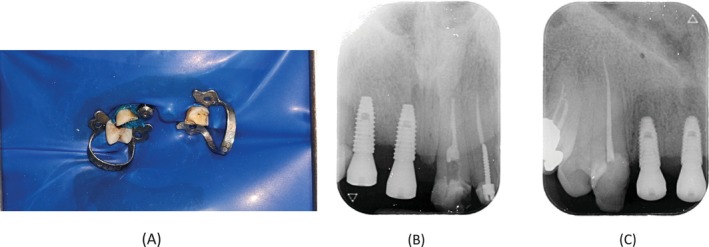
(A) Rubber dam placement during the retreatment session. (B) Final radiograph showing tooth #9 with temporary filling. (C) Final radiograph showing tooth #6 with temporary filling.
